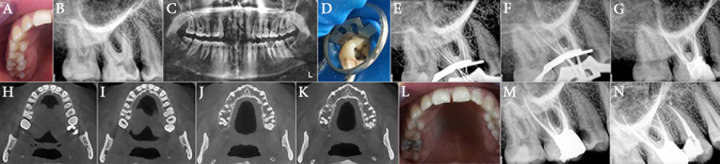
A)* Preoperative photograph; *B)* Preoperative periapical radiograph; *C)* Panoramic view; *D)* Clinical photograph of the access cavity showing four root canals; *E)* Initial files; *F) *Master cones; *G)* Post-obturation periapical radiograph; *H to K)* CBCT images show four root canals in right maxillary first molar; *L)* Photographic view; *M)* Post-restoration radiograph of the tooth; *N)* Six-month follow-up radiograph
